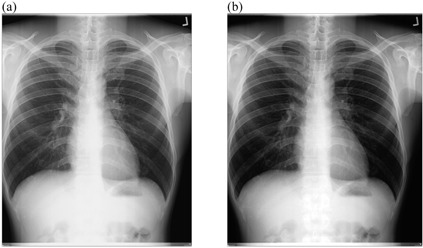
High kVp chest radiograph: (a) original image and (b) processed image by pixel adjustment and histogram equalization.
